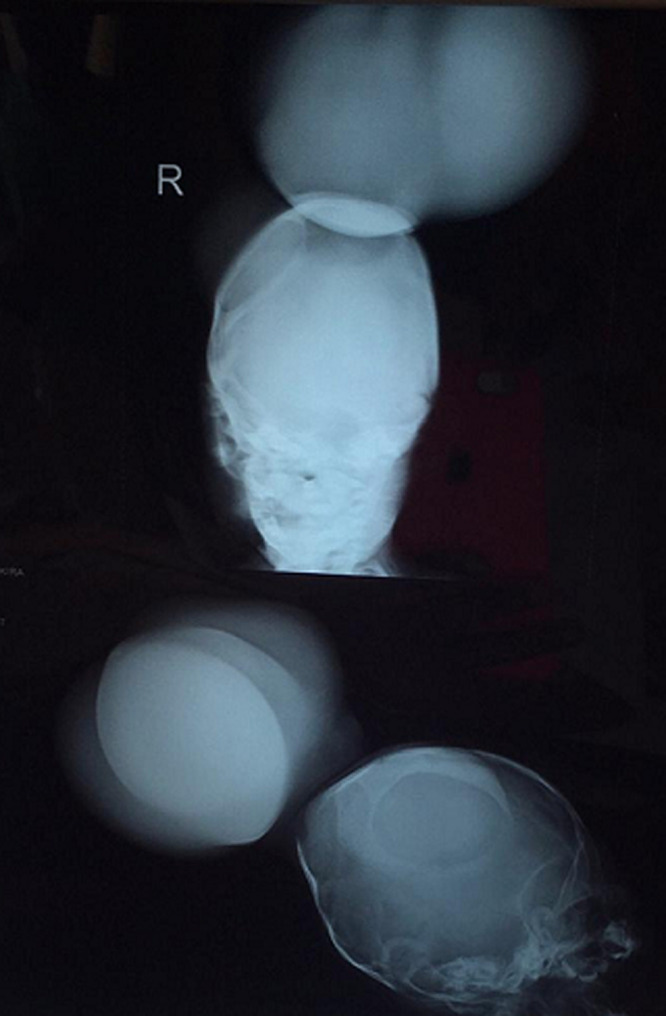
radiographie du crane incidence face et profil montrant une ouverture sur os occipital
